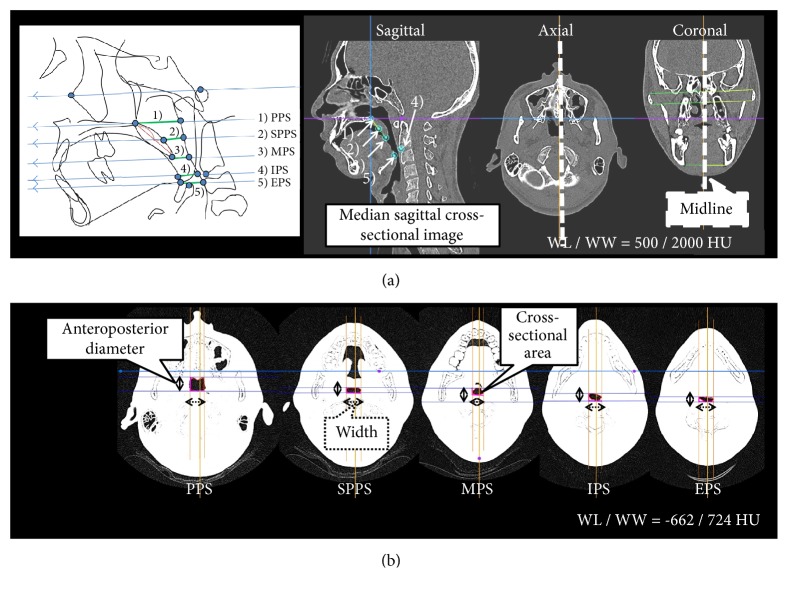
The measurement variables. (a) Midline and median sagittal cross-sectional images. (b) The five measurement sites of the upper airway on a multiplanar reconstruction (MPR) image. The multislice computed tomography data were imported into OsiriX ver. 3.9, with the window level/window width (WL/WW) set to the CT-bone value of 500/2000 HU. Slice datasets were coordinated as follows: (1) the midline of a midpoint of two straight lines, connecting the uppermost and lowermost ends of the bilateral zygomatic process observed in the coronal section, and a straight vertical line parallel to that midpoint were drawn; (2) the median sagittal cross-sectional image was a sagittal section sliced by the midline, and the median sagittal and axial cross-sections were set to the posterior edge of the maxillary bone (PNS); (3) five distance points were established on the MPR image by verifying on the median sagittal, axial, and coronal cross-sectional images; and (4) the WL/WW was set to the airway component value of -662/724 HU, and the anteroposterior diameter, width, and cross-sectional area at the five distance points were measured on the axial cross-sectional images. Abbreviations: EPS, epiglottic pharyngeal space; IPS, inferior pharyngeal space; MPS, middle pharyngeal space; PPS, palatal pharyngeal space; SPPS, superior posterior pharyngeal space.
